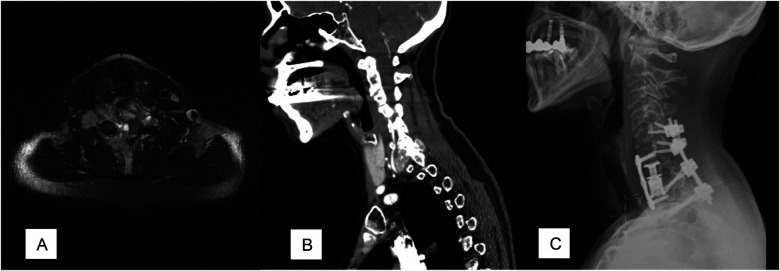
(A) Initial presentation of a 67-year-old female patient with recurrent clear cell chondrosarcoma of the cervical spine. MRI shows an enhancing lesion disrupting C7 and invading the spinal canal; (B) angio-CT shows the anterior dislocation of the right vertebral artery and the tumoral matrix characterizing the lesion; (C) postoperative X-rays. The patient underwent GTR and posterior decompression, followed by circumferential stabilization. This reconstruction comprised an anterior cervical approach using an expandable titanium cage with C6–T1 plating, supplemented by posterior instrumentation spanning C5 to T2.
